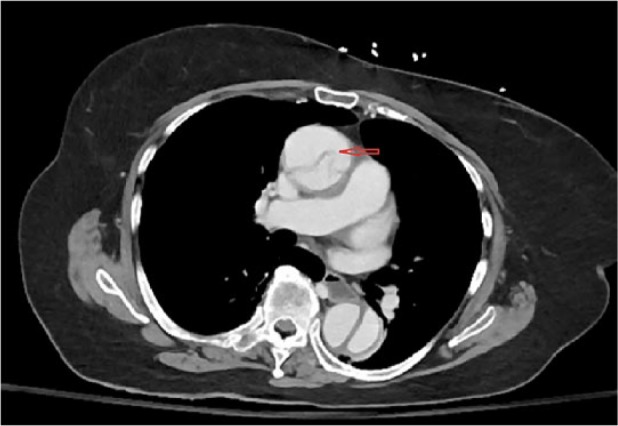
Arrow indicating to dissection flap in the ascending aorta on the contrast enhanced CT.
